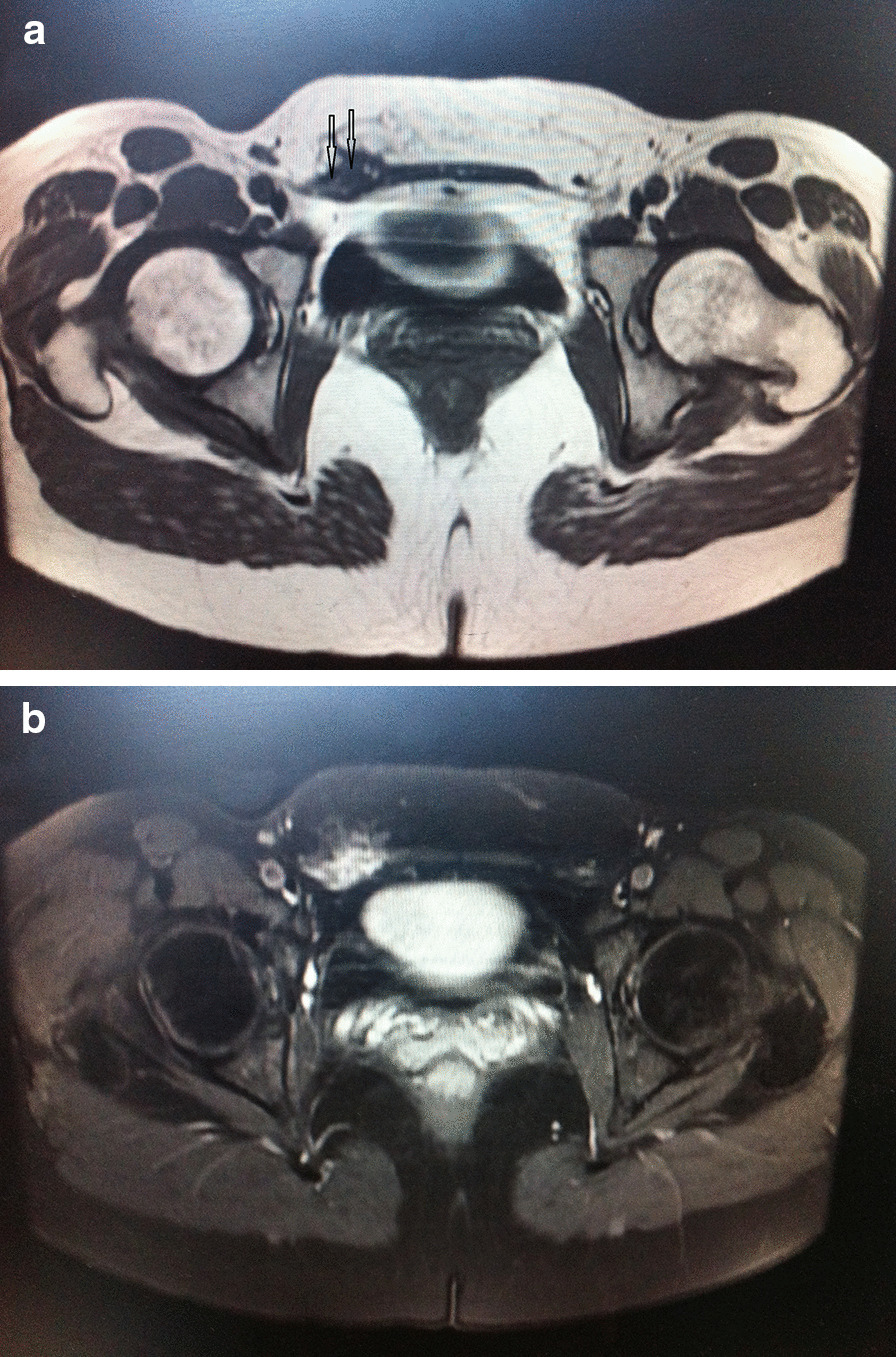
The magnetic resonance imagining reveals an inguinal mass, isointense with muscle, which infiltrate the edge (arrow) of the abdominis rectus muscle, in Axial T1-weighted imagine (A). Axial T2-weighted image, obtained at the same level (B)
